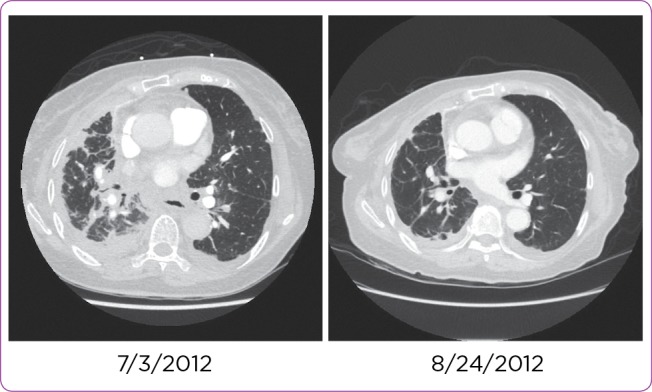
CT scans of the patient in Case Study 1 before targeted therapy (left) and showingresponse within 1 week of starting ceritinib (right).
